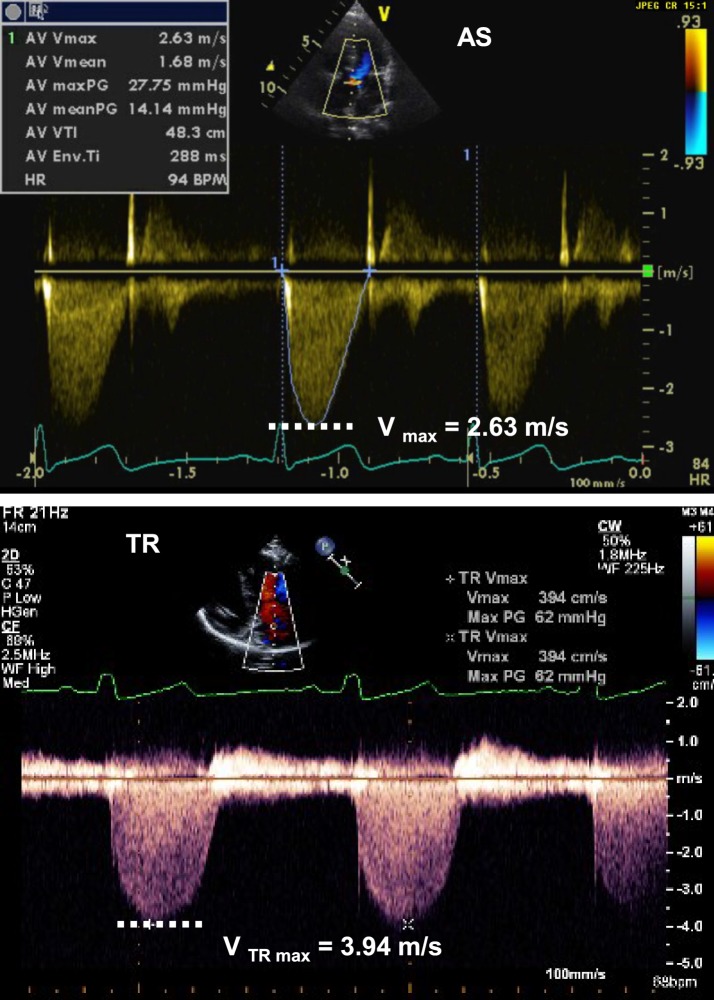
Spectral Doppler tracing (picture above) from aortic valve stenosis (AS) showing *V*_max_ of 2.63 m/s, allowing for estimation of a pressure gradient across the valve of 28 mmHg, consistent with moderate aortic valve stenosis. Spectral Doppler tracing (below picture) from tricuspid regurgitation (TR) showing Vmax of 3.94 m/s, allowing for estimation of a SPAP of 62 mmHg + RAP. RAP, right atrial pressure; SPAP, systolic pulmonary artery pressure.
